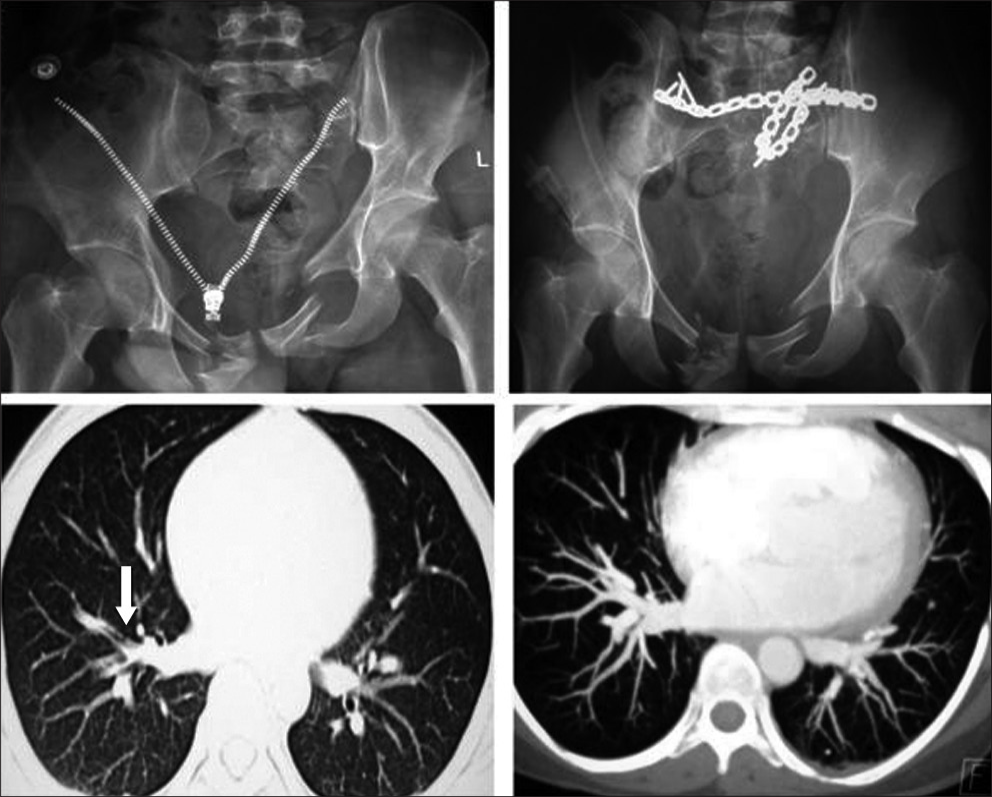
Radiograph showing a vertically unstable pelvic injury in a 22-year-old male. The fracture was stabilized by open reduction and internal fixation with recon plates. Postoperative computerized tomographic pulmonary angiographic films show embolus in right lung field (arrow), compared to normal pulmonary arterial appearance in film on right side.
